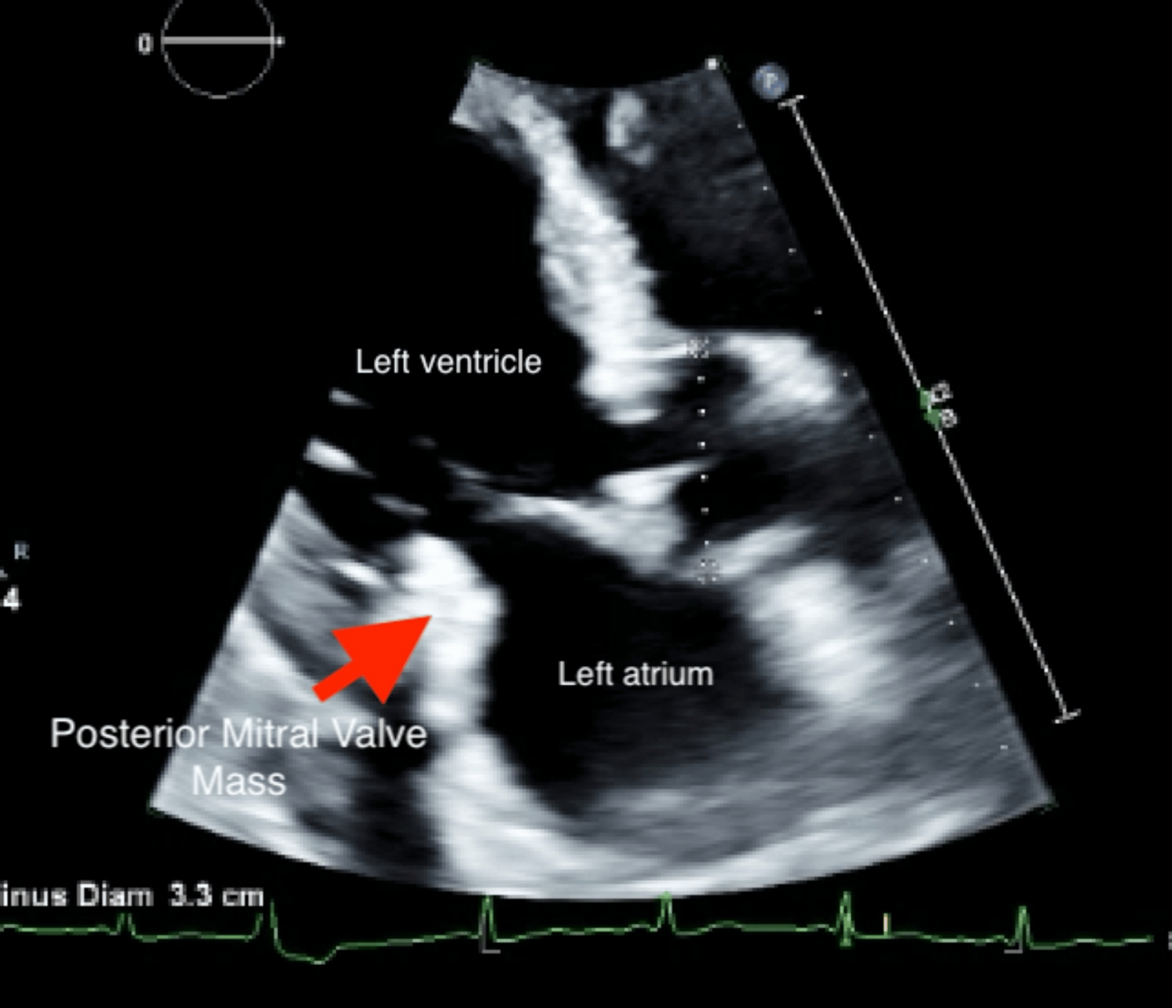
Transthoracic echocardiogram revealing a left ventricular ejection fraction of 50-55% with a large mobile echogenic frond-like irregular multi-lobular mitral valve mass (red arrow) on the posterior valve leaflet with no mitral regurgitation noted.
